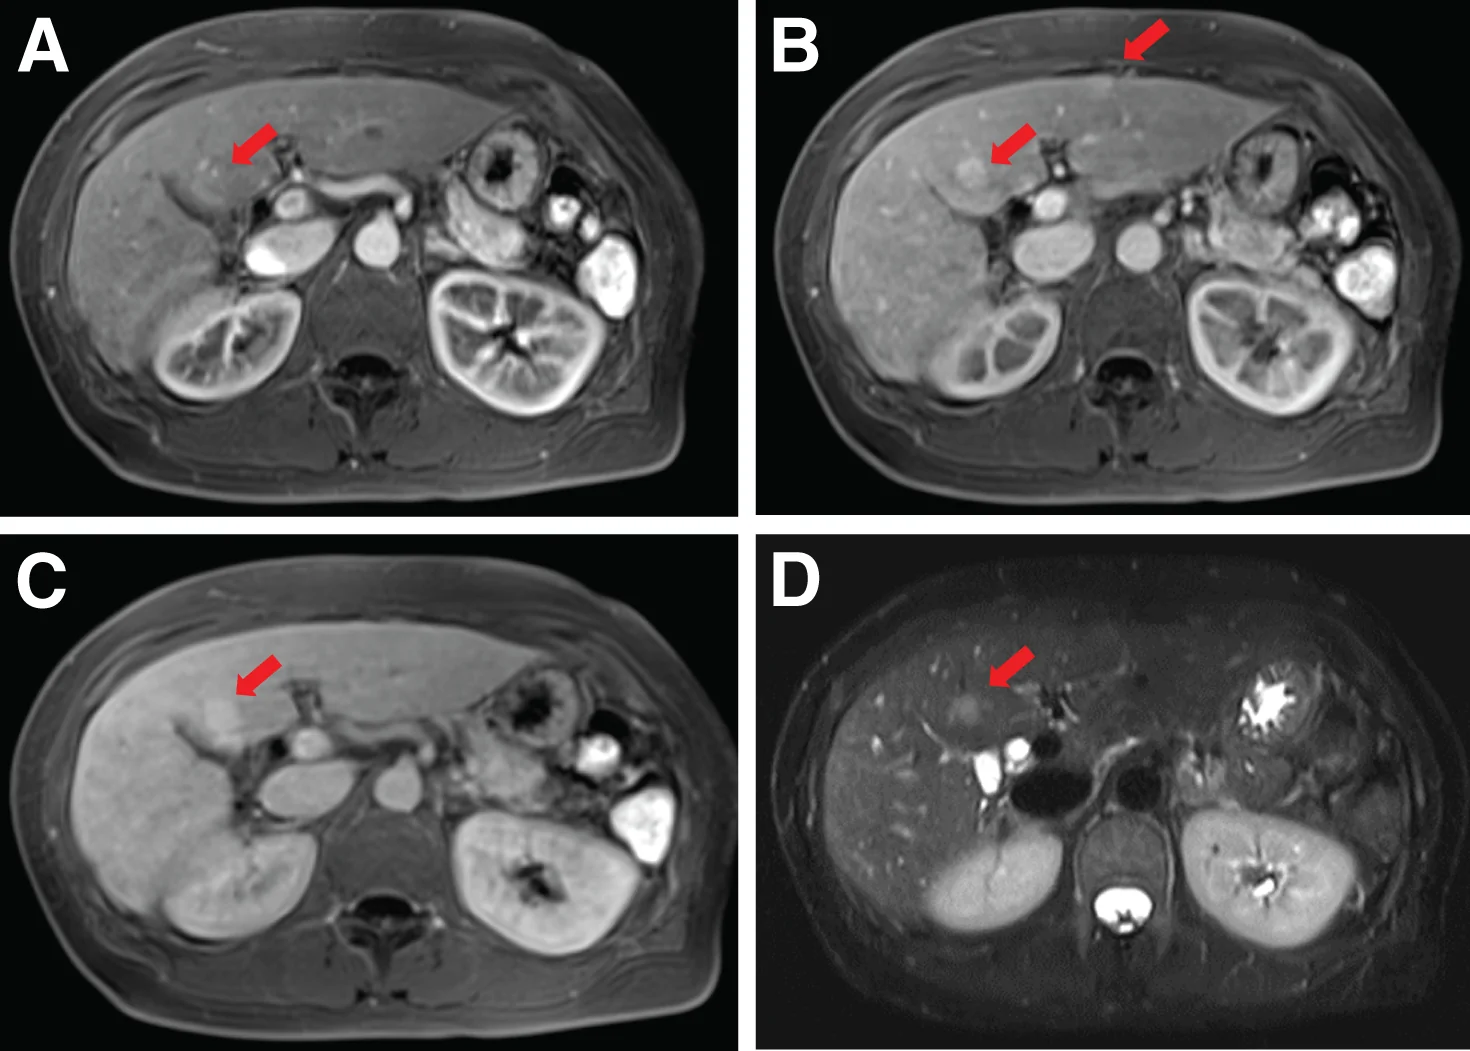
EOB-MRI showing hepatic lesions in segments IV and III during T-DM1 therapy. (A) Arterial phase image showing faint arterial phase hyperenhancement of the lesions. (B) Portal venous phase and (C) transitional phase images showing persistent hyperintensity. (D) Hepatobiliary phase image showing mild hyperintensity of the segment III lesion and approximately isointensity of the segment IV lesion, without definite hepatobiliary phase defects. EOB, gadolinium-ethoxybenzyl-diethylenetriamine pentaacetic acid-enhanced; T-DM1, trastuzumab emtansine
